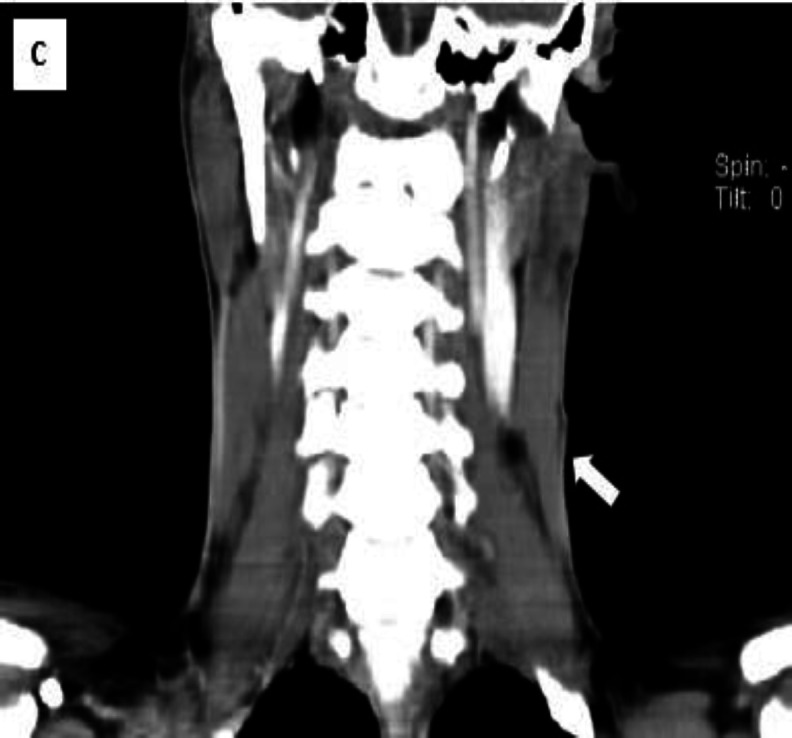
Spiral neck CT scan, coronal view. The tract extended superficially through SCM (the arrow)
